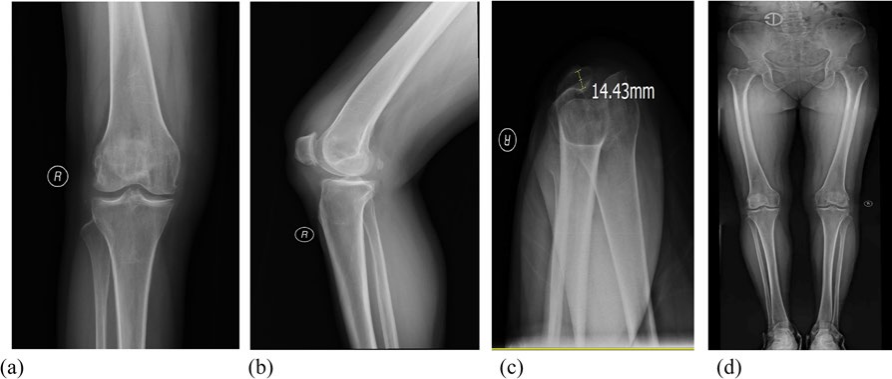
a Anteroposterior plain film of the knee joint (weight-bearing position), b Lateral plain film of the knee joint (weight-bearing position), c Axial flat patellar film (weight-bearing position), d total-length plain film of the lower extremity (weight-bearing position)
